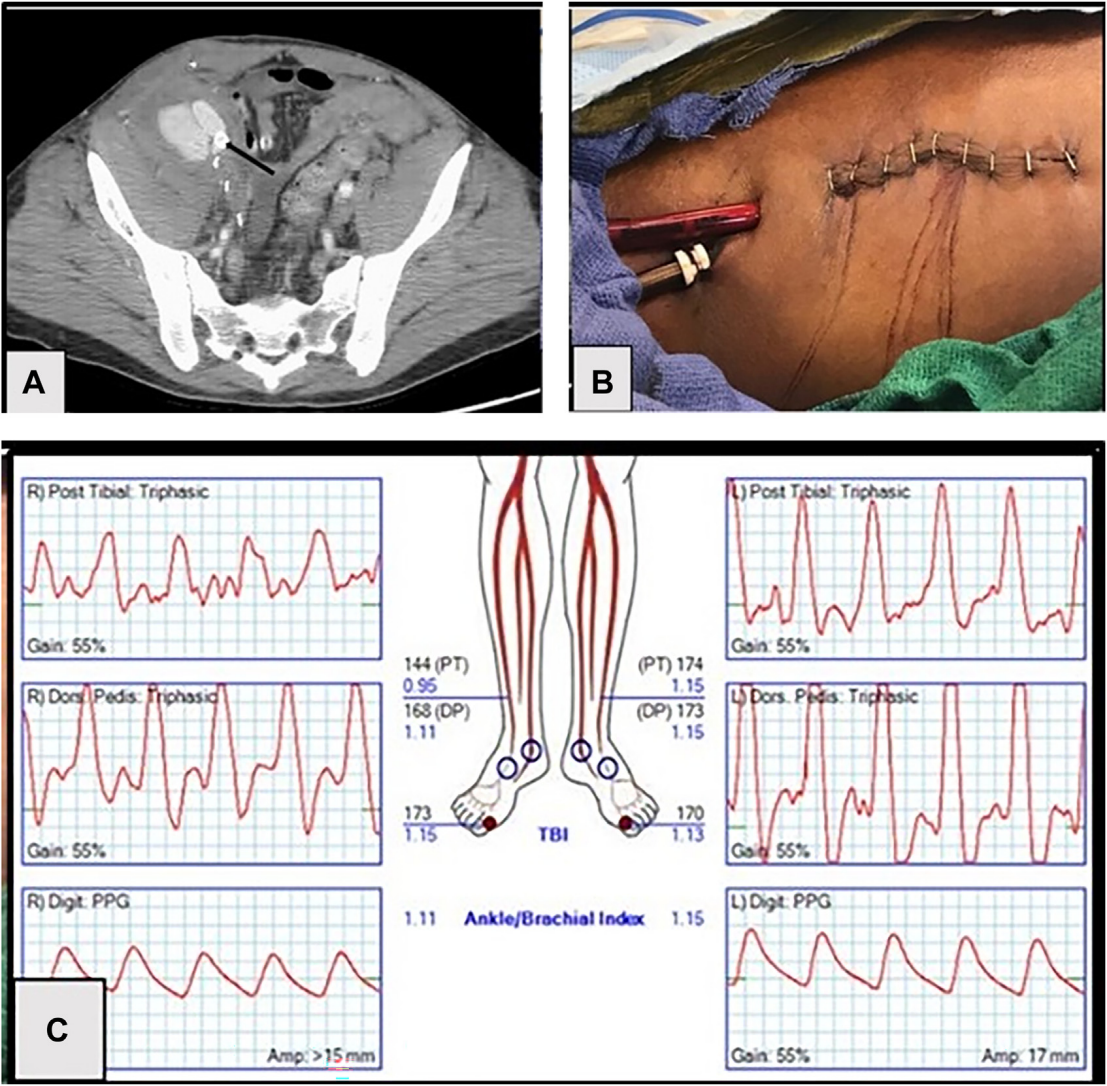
(A) Contained rupture of infected external iliac artery pseudoaneurysm with previously placed covered stents visible (arrow). (B) Extracorporeal membrane oxygenation cannulation of the superficial femoral artery and vein through a separate upper thigh incision. (C) Postoperative Doppler study demonstrating normal lower extremity waveforms and ankle/brachial index. (DP, dorsalis pedis; PPG, photoplethysmography; PT, posterior tibial; TBI, toe/brachial index.)
